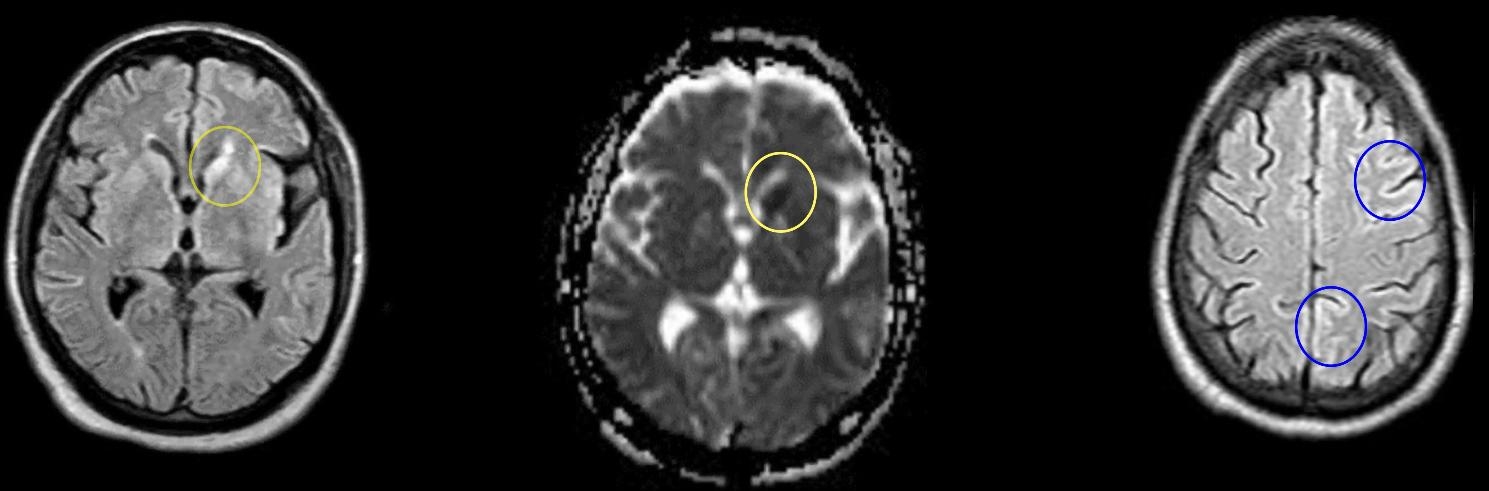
Brain MRI. The brain MRI revealed widespread atrophy, with both sides showing increased signal intensity in the caudate nucleus. The left caudate nucleus (marked yellow) was more prominently affected than the right, and there were similar changes observed in the thalamus on both sides. Additionally, signs of cortical ribboning were seen (marked blue), with the left side being more affected compared to the right
